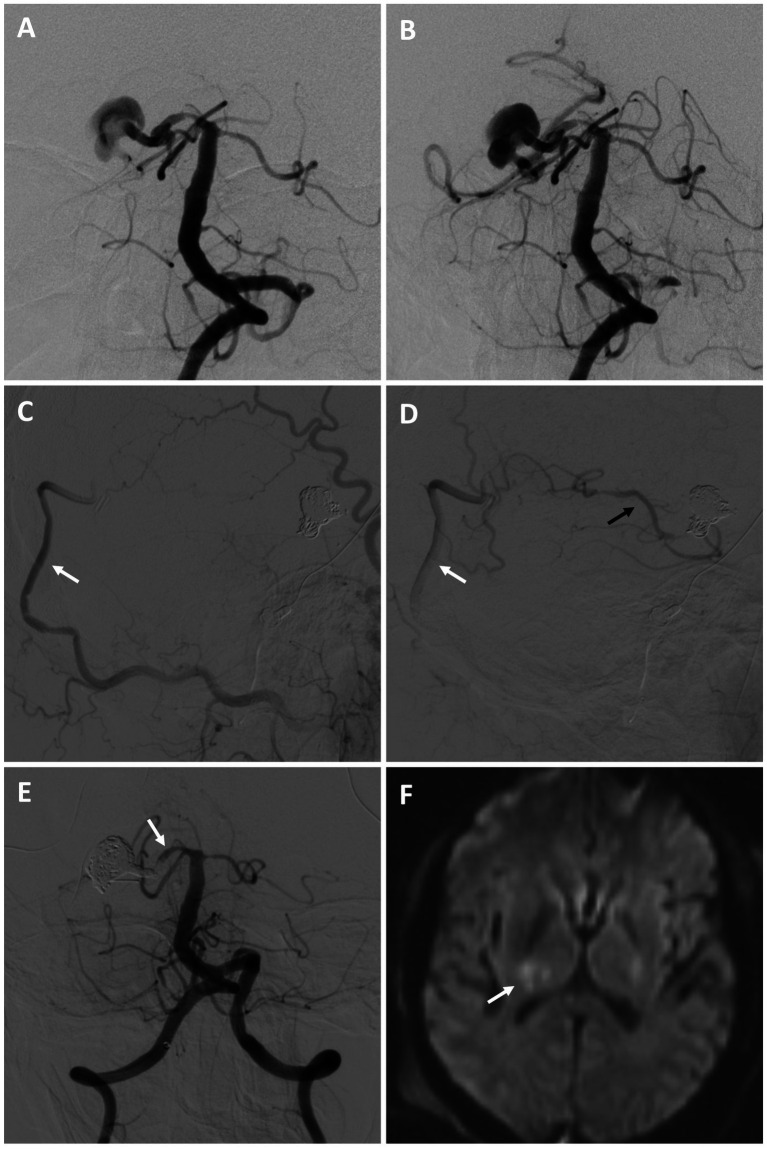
Patient #10. A 53-year-old male was referred for a large right fusiform P2 aneurysm with a proximal dysplastic P1 vessel [(A,B) AP angiogram of the left vertebral artery]. A prior attempt at flow diversion was aborted due to unfavorable anatomy. He underwent a right OA to P4 bypass with a DLCFA interposition graft, followed by a coil embolization/vessel sacrifice of the right P2 aneurysm (keeping the proximal right P1 patent) after BTO [(C,D) serial lateral images from a right external carotid artery injection demonstrating patency of the bypass (white arrows) and backfilling of the PCA territory (black arrow)]. The right P1 was kept open to preserve perforator flow [(E) AP angiogram of the right vertebral artery (arrow highlights right P1)]. Post-operatively he experienced a small right thalamic stroke [(F) axial diffusion-weighted MRI, arrow highlighting stroke], likely from perforators off the severely diseased and sacrificed P2 segment, as well as a small right occipital stroke of unclear etiology (not shown). He had left mild hemiparesis and a partial left hemianopsia. This significantly improved on 9-month follow, where he was living at home and performing all activities of daily living independently.
